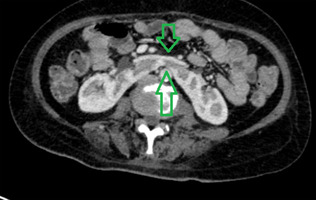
computed tomography scan of coronal section: green arrows indicate the area of fused left and right kidney forming a horseshoe kidney formation retroperitoneally near the lumbar spine
